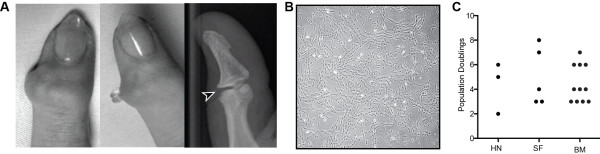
Sample collection, cell growth, and multipotential stromal cell characterization. (A) Distal interphalangeal joint with osteoarthritis and an acute Heberden’s node (HN) with extrusion of gelatinous material and plane radiograph demonstrating visible joint space (arrowhead) indicating preservation of articular cartilage at clinical presentation. (B) Example of multipotential stromal cell-like colony grown from gelatinous cyst material and (C) population doublings of HN cells between passages 0 and 1 compared with synovial fluid (SF)- and iliac crest bone marrow-multipotential stromal cells. BM, bone marrow.
